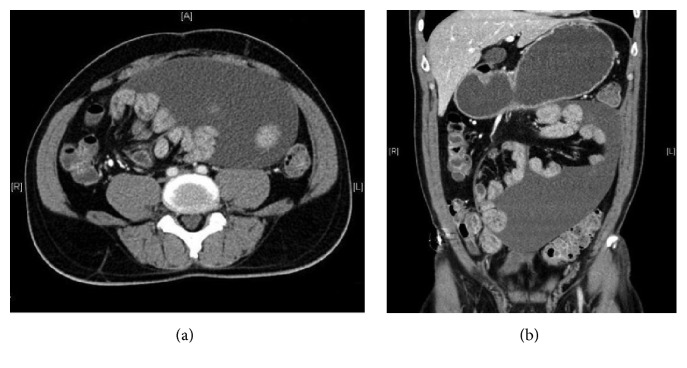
Contrast-enhanced CT in (a) axial and (b) coronal sections showing encasement of the mildly dilated small bowel loops within a membranous sac with thin wall. Moderate amount of free fluid is noted within the sac.
